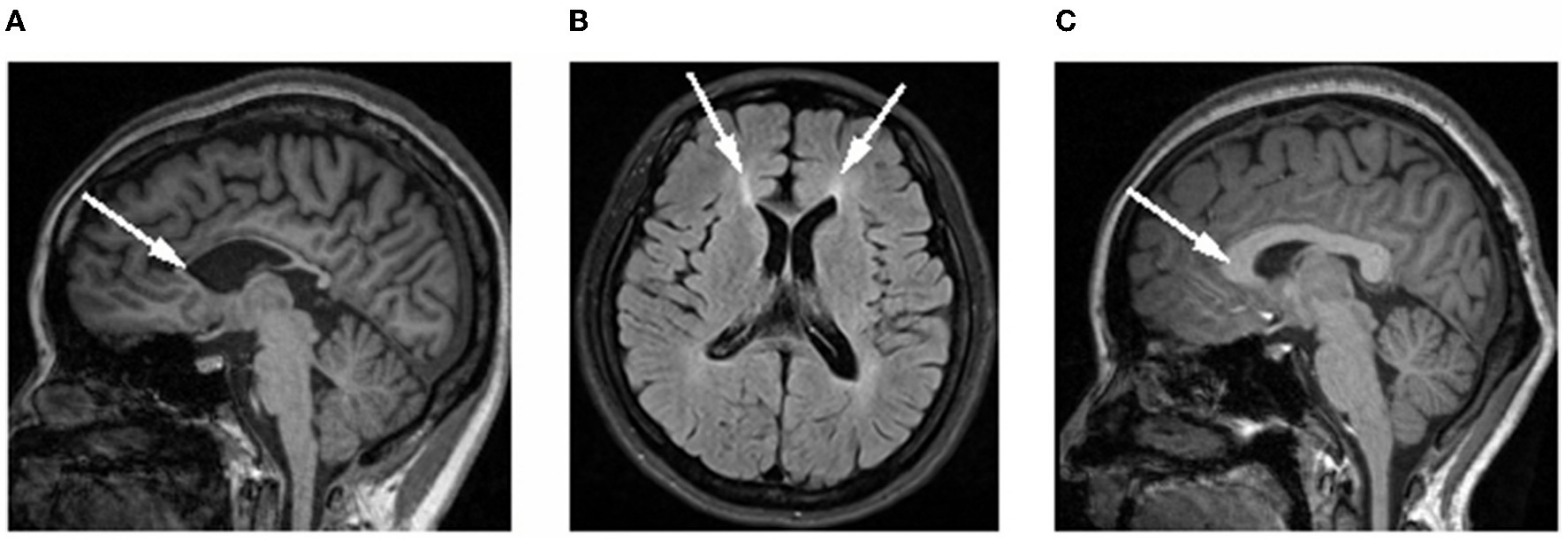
Brain MRI in the patient and a healthy control subject (the same age girl). (A) Sagittal T1-weighted image showing thin corpus callosum (white arrow) in the patient, compared to normal corpus callosum morphology in a control subject. (B) Axial FLAIR image showing typical “ears of the lynx” sign (white arrow). (C) Brain image in the healthy control subject.
